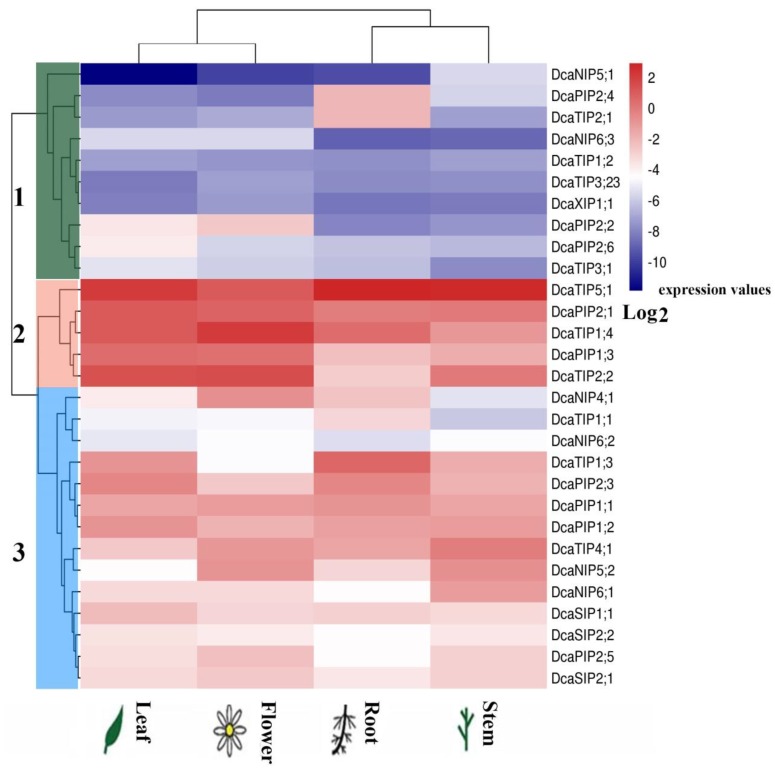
The qRT-PCR analysis of expression profiles in different tissues. Expression values of DcaAQP genes were calculated by comparative CT(2^−ΔΔCT^) method. A heat-map shows the hierarchical clustering of relative expression of all DcaAQP genes across in four different tissues analyzed. The vertical color scale at right of the image represents log_2_^expression values^: red indicates a high level and blue represents a low of transcript abundance.
